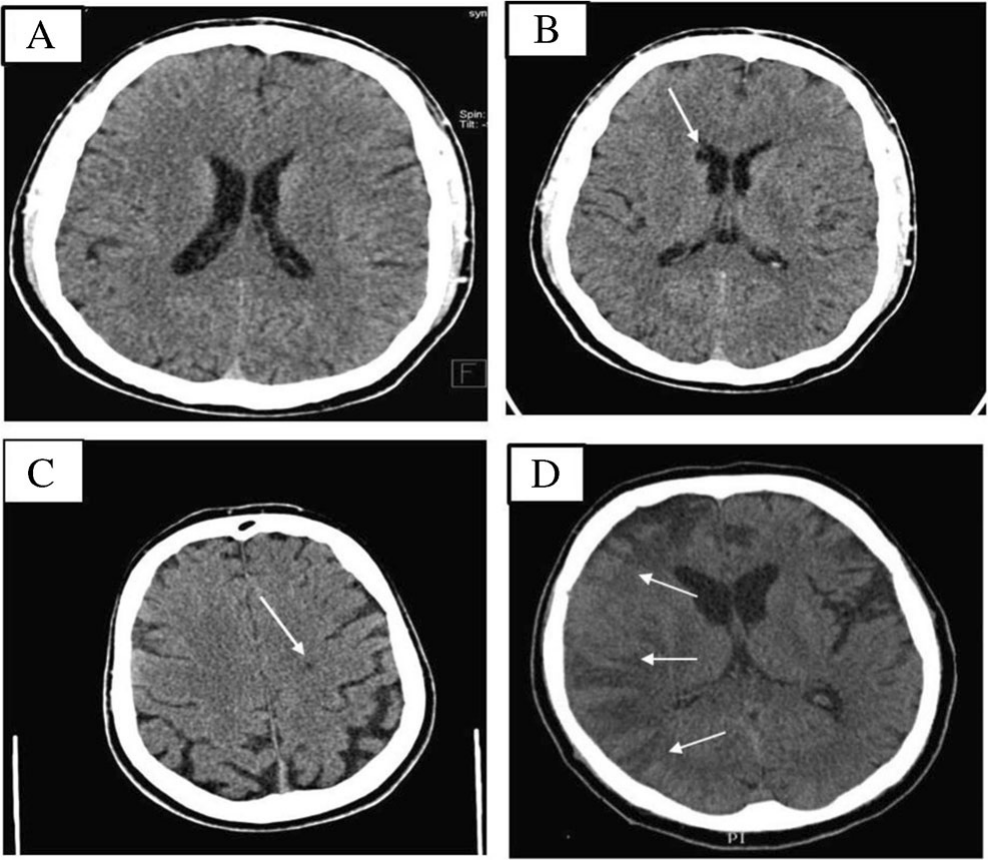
Non-contrast enhanced cerebral CT scan of patients after TALV lead implantation: a no abnormality; b 6 mm lacuna in the right-sided nucleus caudatus, c 4 mm hypodensity in left-sided centrum semiovale, d middle cerebral artery occlusion with right-sided fronto-temporo-parietale extension
